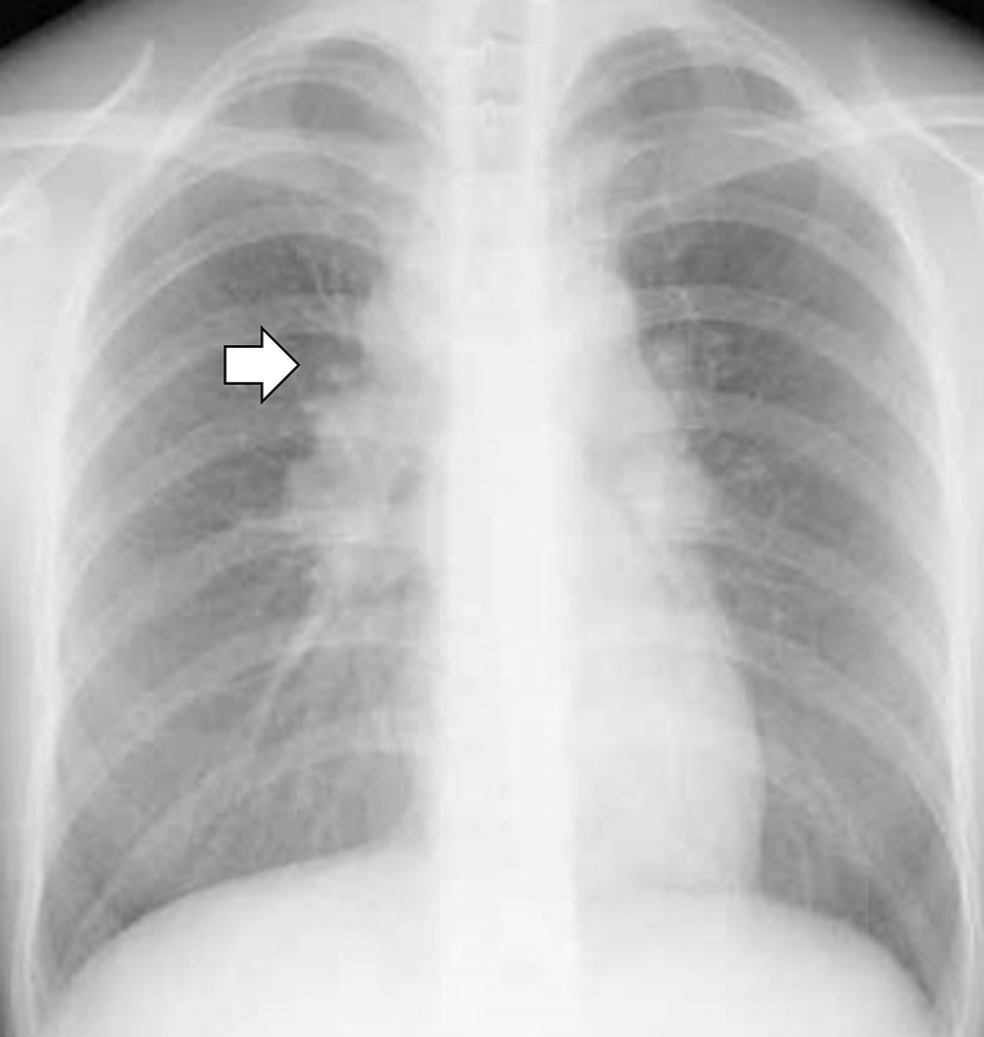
Chest radiography revealing bilateral hilar enlargement at the first visit.
